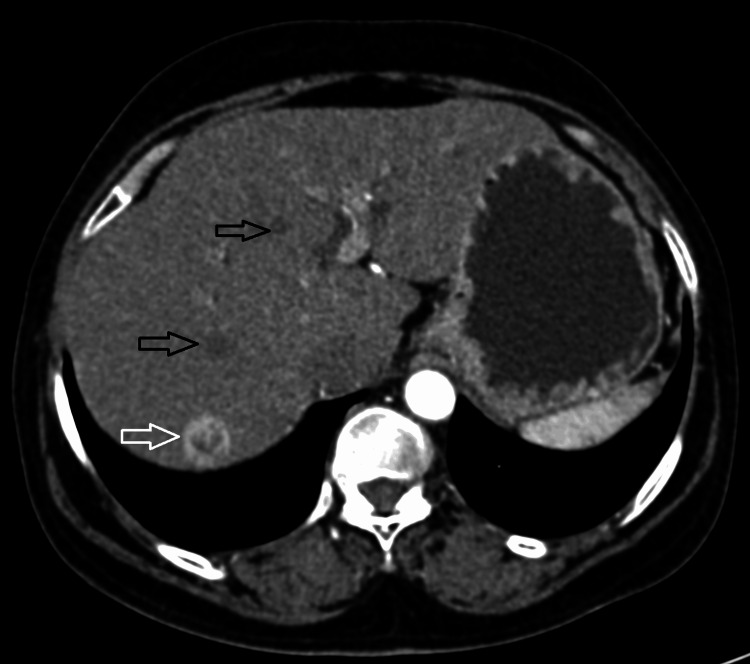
CECT image of arterial phase in axial section, showing hypervascular lesion in segment VII of the liver, likely hypervascular liver metastasis (white arrow). Few hepatic cysts were also noted (black arrows) CECT: contrast-enhanced computed tomography
